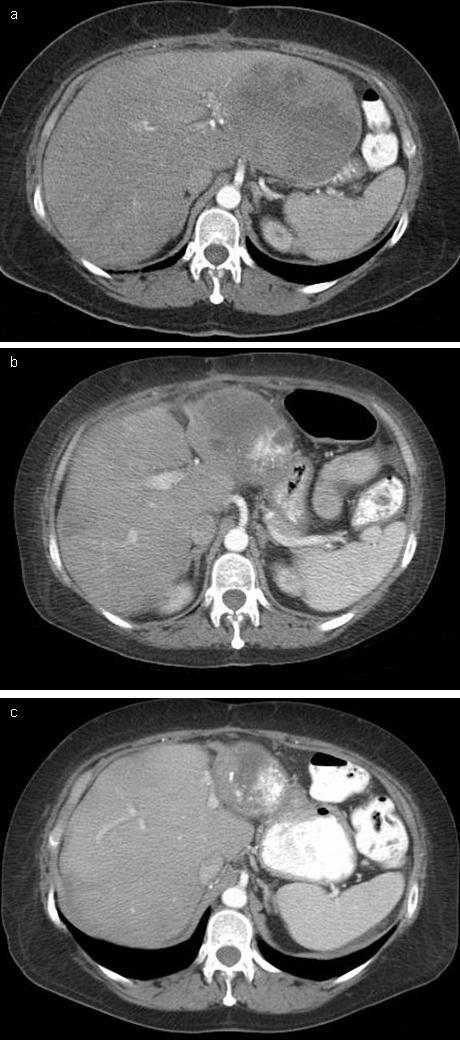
Computed tomography scan of metastatic carcinoid prior to TACE (A), four months after TACE (B), and eight months after TACE (C) showed marked reduction in hepatic tumor burden.
